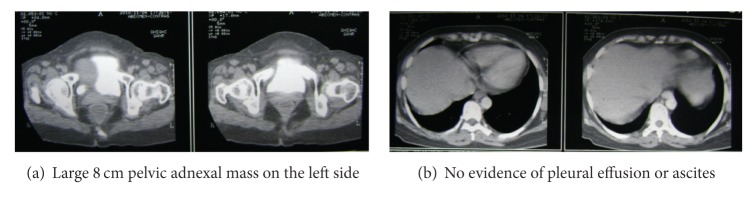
CT scan after 3 cycles of chemotherapy.
